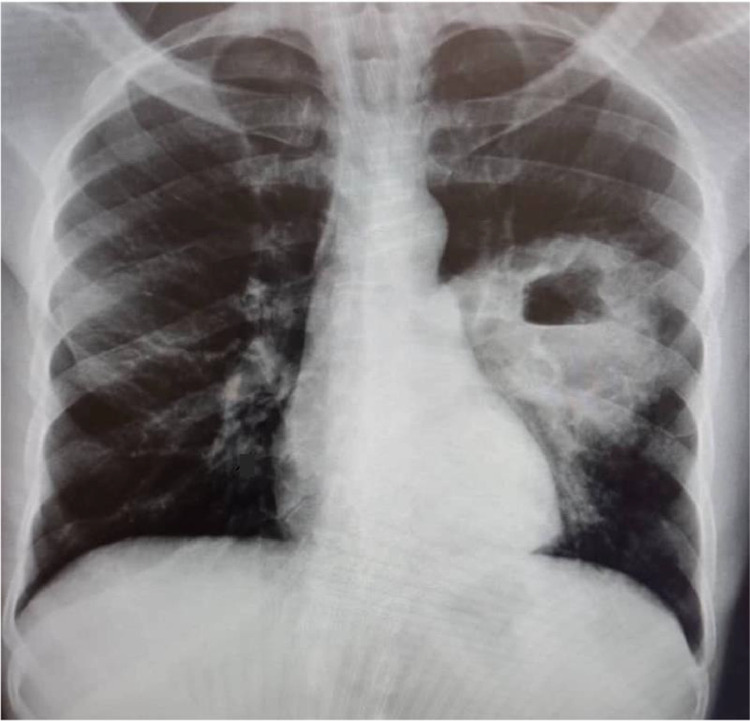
PA Erect Chest X-ray shows left middle zone thick-walled cavity with an internal air-fluid level and surrounding consolidation.
